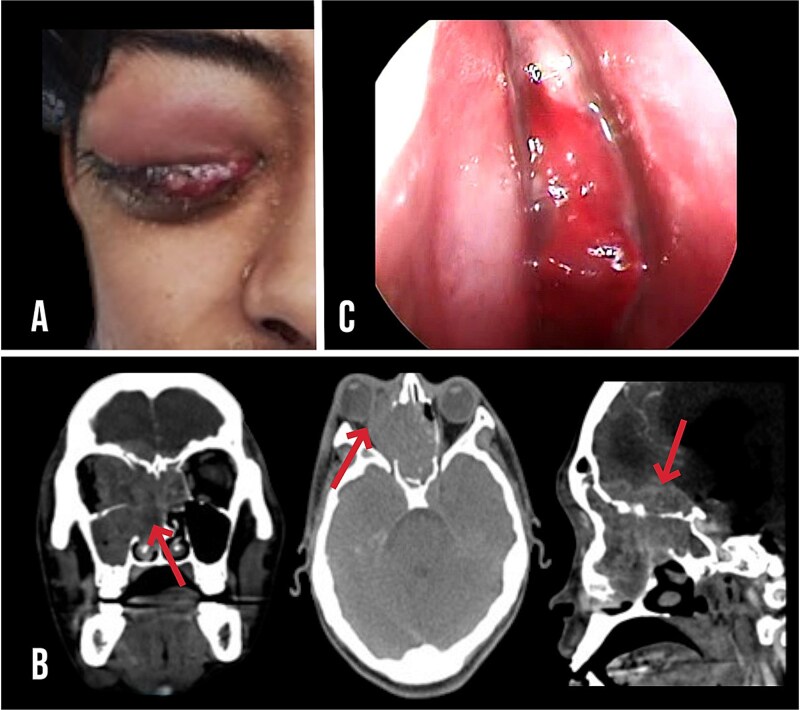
(A) 17-year-old male presenting with proptosis, redness, and swelling of the right eye. (B) CT in coronal, axial and sagittal view, showing a tumour occupying the right frontal and maxillary sinuses, extending into the orbit and causing osteolytic destruction at the anterior skull base with signs of intracranial invasion, respectively (red arrow). (C) Nasoendoscopic image of the tumour at the level of the right inferior turbinate, which was subjected to biopsy.
